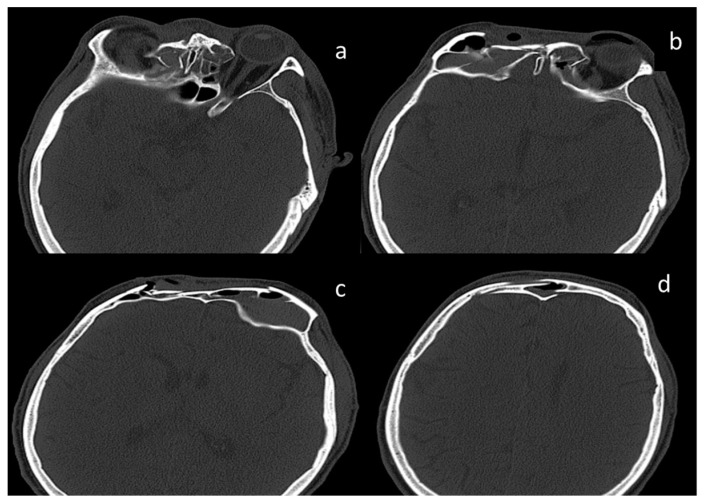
CT images, bone window, axial sections. (a) Naso-orbito-ethmoid (NOE) fracture with major depression and ethmoid hemosinus, (b,c) comminuted fracture of the anterior frontal sinus walls with frontal hemosinus, subcutaneous emphysema, and left pneumo-orbitis, (d) displaced posterior frontal sinus wall fracture.
